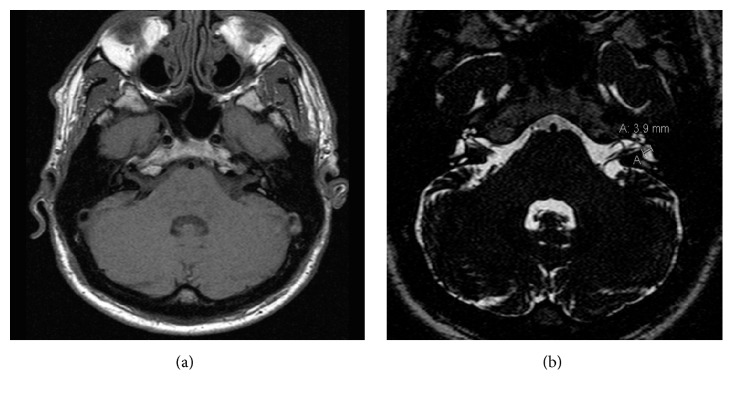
MRI. TSE T1 (a) and T2 WIs (b) images. The area of altered signal intensity in the left utricle is clearly defined while no abnormalities are seen in the right utricle.
